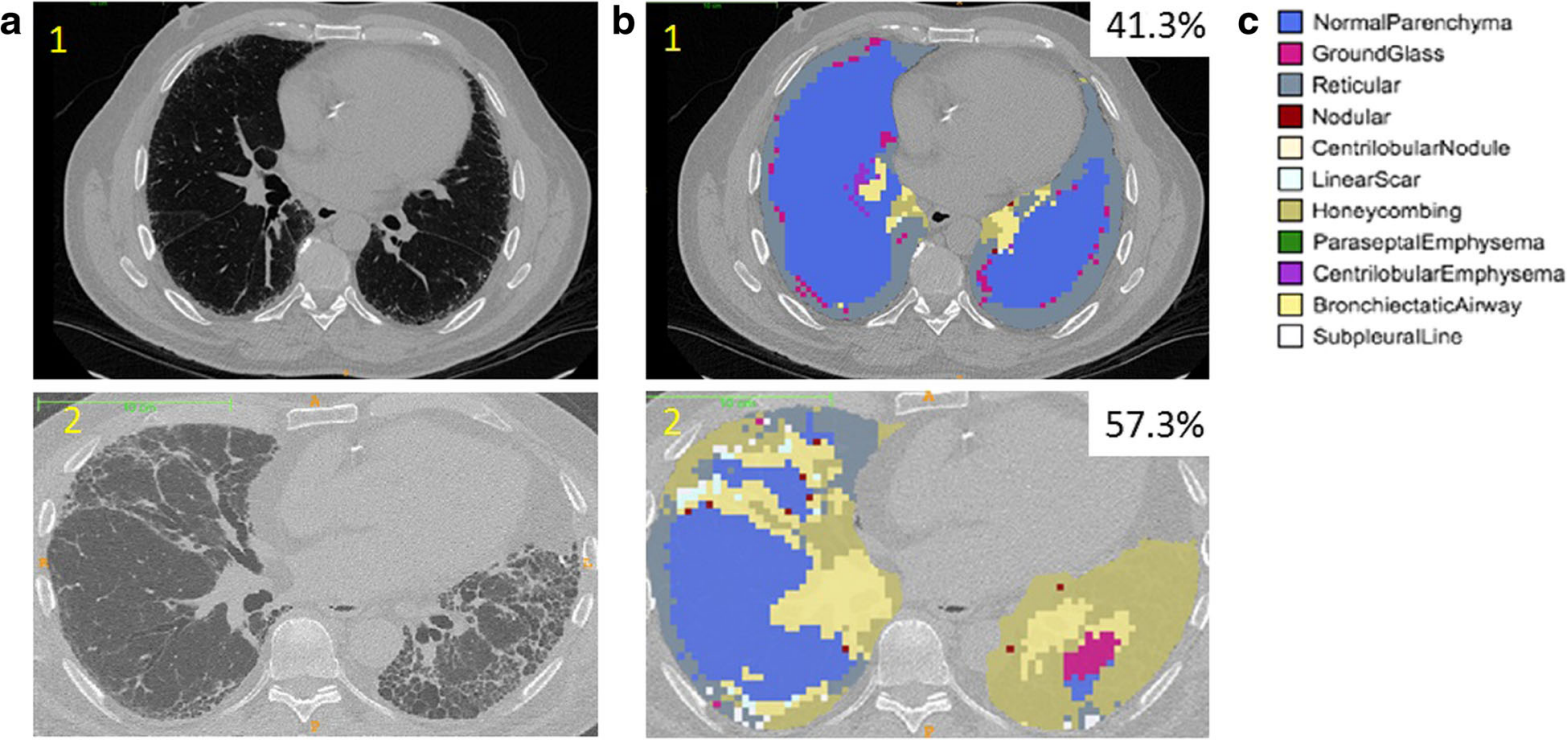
a Representative CT images from subjects with less severe IPF (patient 1) and more severe IPF (patient 2). b Overlay of categorization of lung parenchyma into radiographic subtypes using the local histogram analysis and distance based analysis for each subject. c Legend for radiographic subtypes
